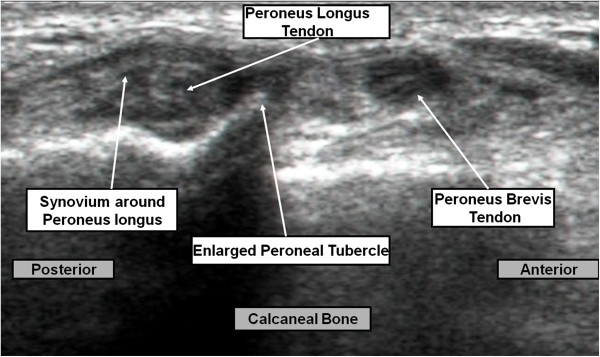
Ultrasound showing hypertrophic peroneal tubercle and the thickened peroneus longus with synovitis around the tendon.
